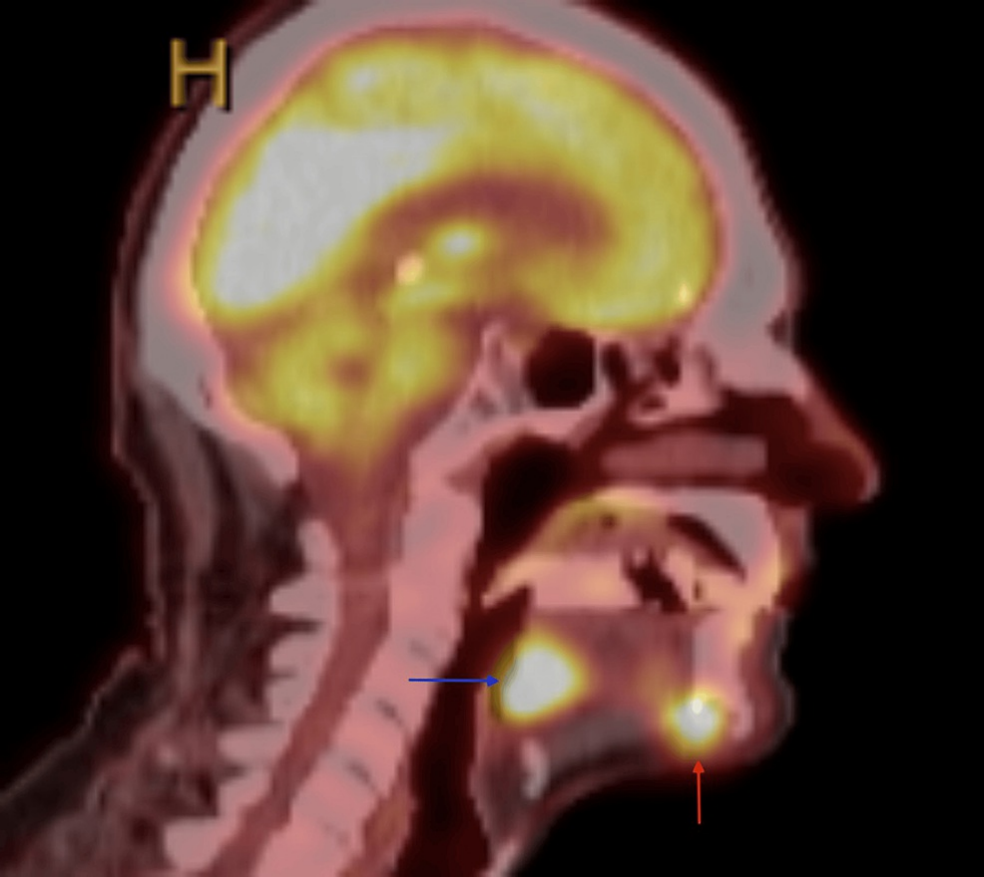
Sagittal PET/CT of Mandibular and Base of Tongue Mass Sagittal PET/CT neck showing FDG avid soft tissue mass in the mandibular symphysis (red arrow) and a left base of tongue mass (blue arrow)
